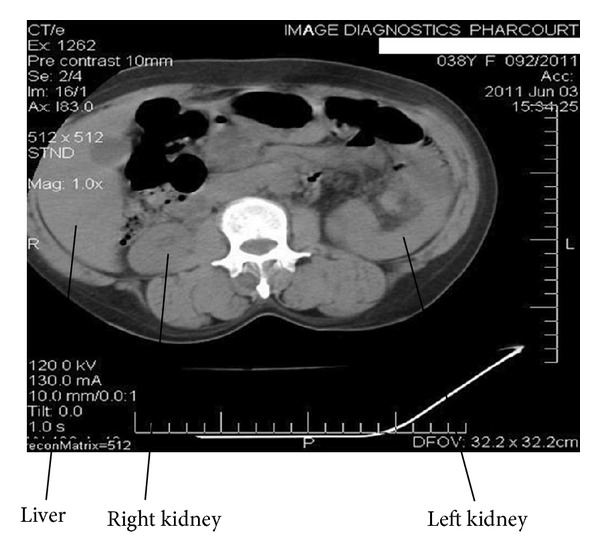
This CT slide shows both kidneys and the liver.
